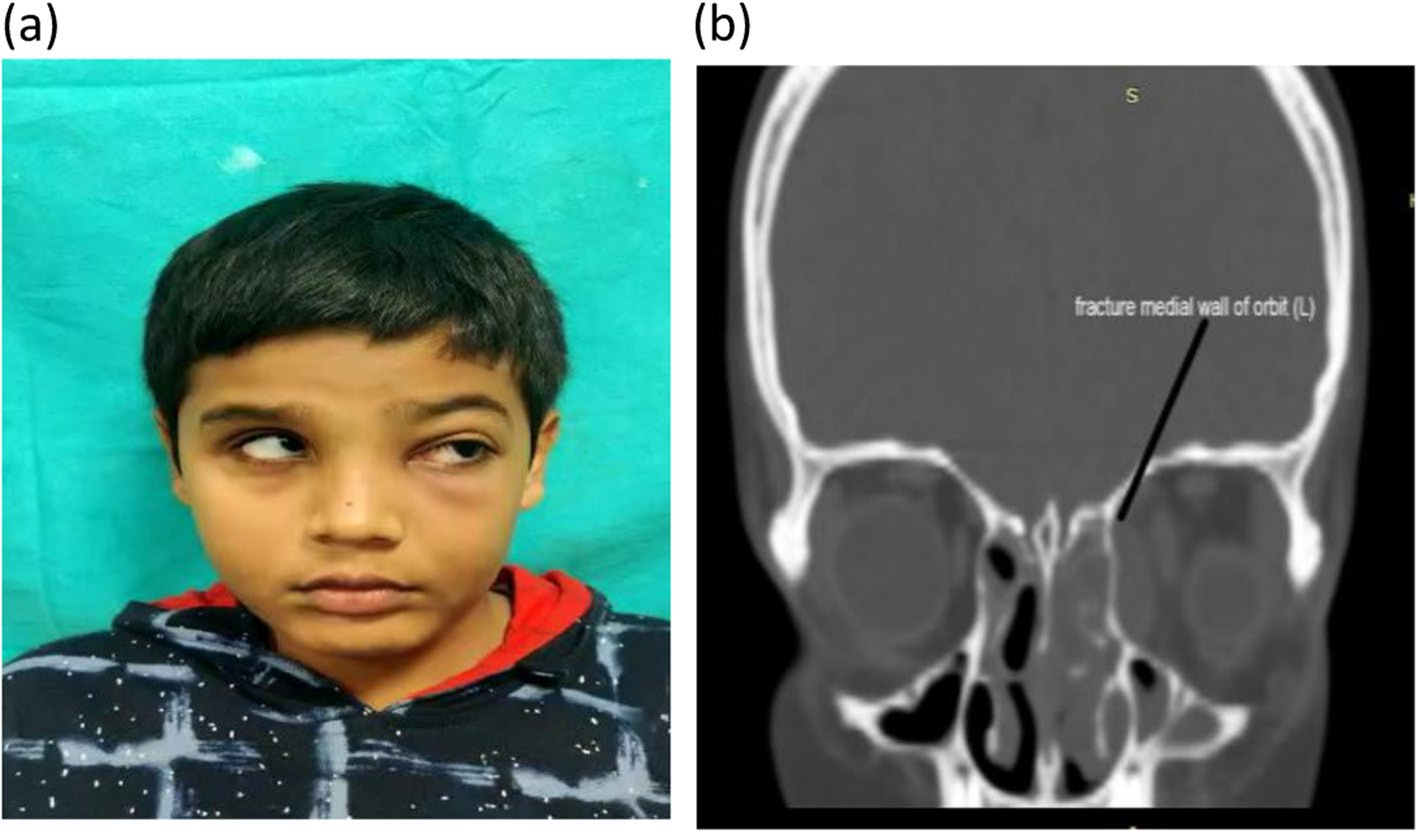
a, b Representative images of case series 2. a (L) periorbital swelling, mild erythema, Proptosis (L) eye, restricted superior gaze in a case of complicated rhinosinusitis with subperiosteal abscess. b CT PNS image showing fracture of medial wall of (L) orbit, subperiosteal hematoma along medial wall of (L) orbit with proptosis, indentation of (L) medial rectus. Hemosinus in (L) ethmoidal air cells and mucosal thickening involving all paranasal sinuses
